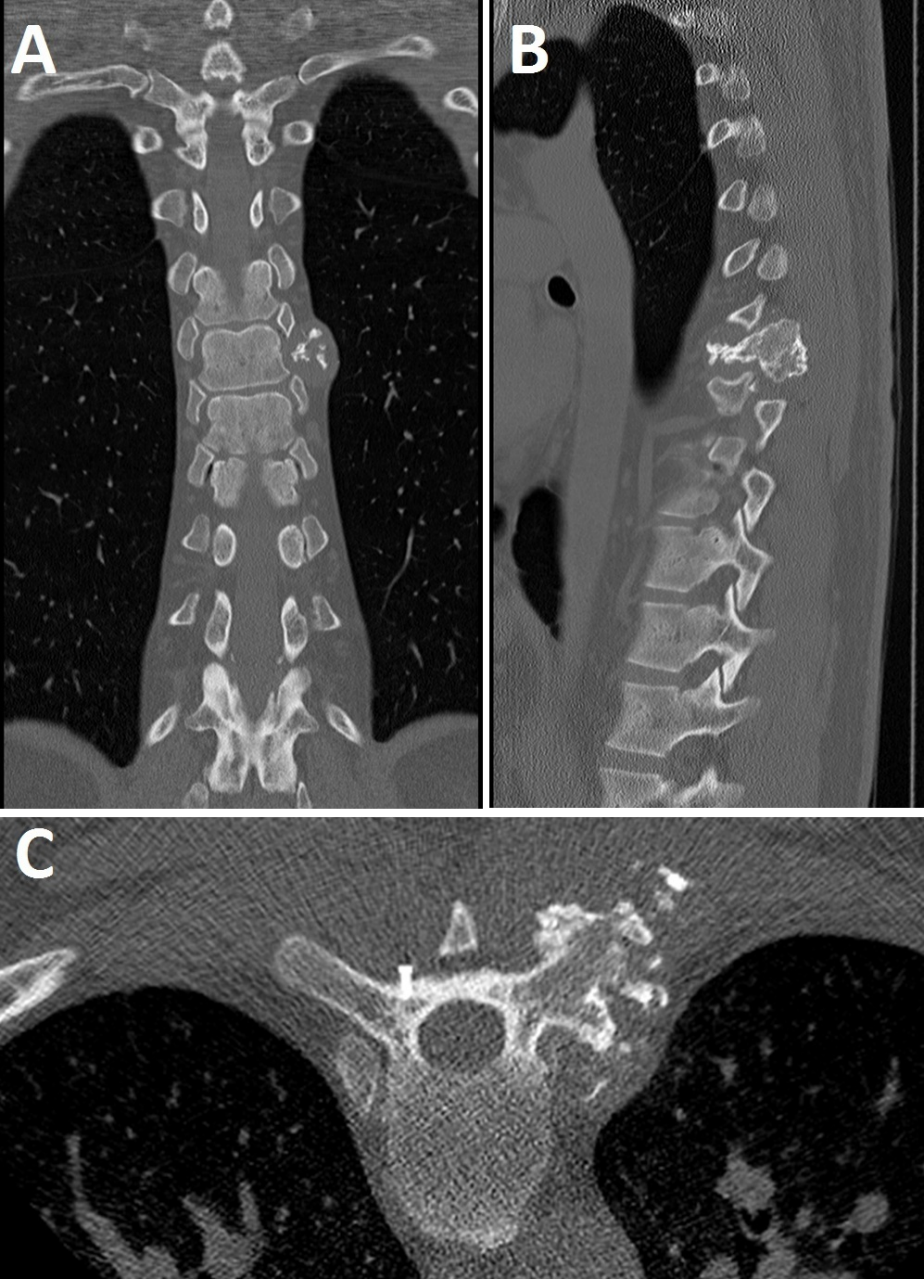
Preoperative CT Imaging Preoperative coronal (A) and sagittal (B) CT images showing a polylobulated mass centered around the left T7 costotransverse joint. A preoperative axial CT image showing fiducial screw placement (C). The fiducial screw is seated within the cortex of the right lamina/transverse junction at the level of the vertebral pedicle, the same level of the involved costovertebral joint contralaterally.
